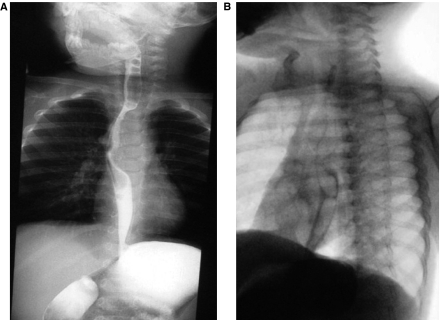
Esophageal fluoroscopy.A:anteroposterior radiograph;B: lateral radiograph.
